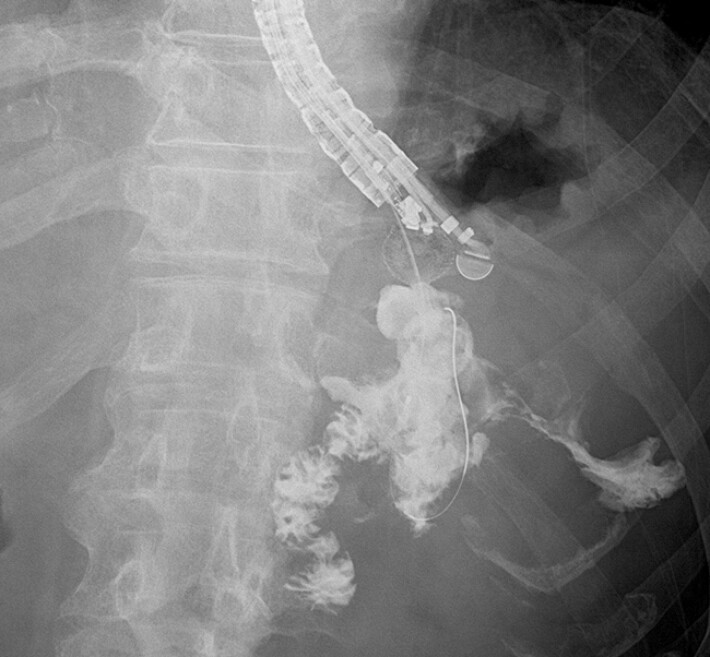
A radiographic image showing contrast injection into the walled-off necrosis through the lumen-apposing metal stent, demonstrating communication with both the small intestine and the colon.
